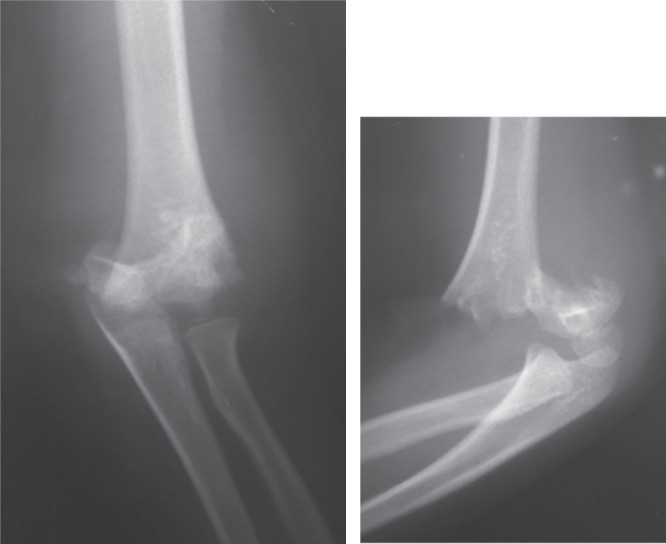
Radiographs showing fracture of the supracondyle of left humerus.
